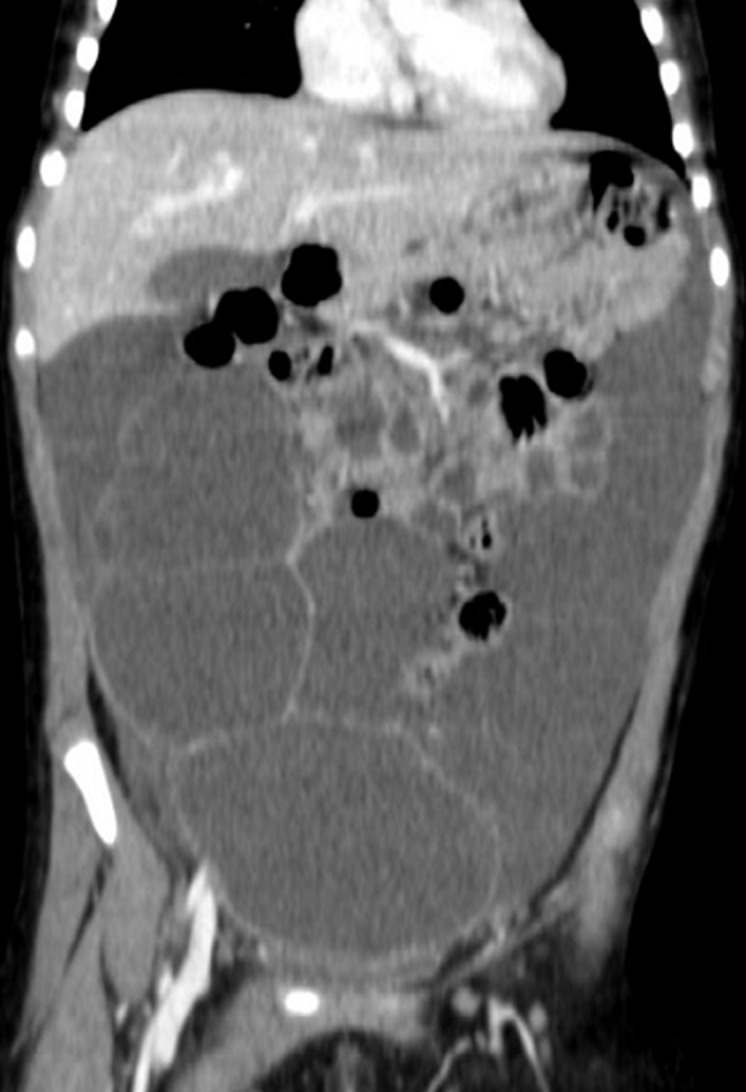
computed tomography image demonstrating a giant multilocular cystic lesion, occupying the entire abdominal cavity laterally
